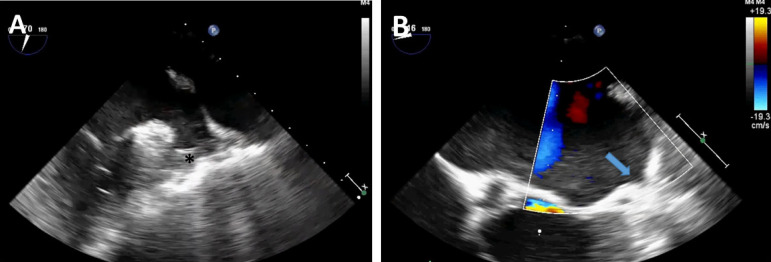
A) Intraoperative transesophageal echocardiography showing left atrial thrombus (asterisk); B) Postoperative result with left atrial appendage exclusion (arrow).
